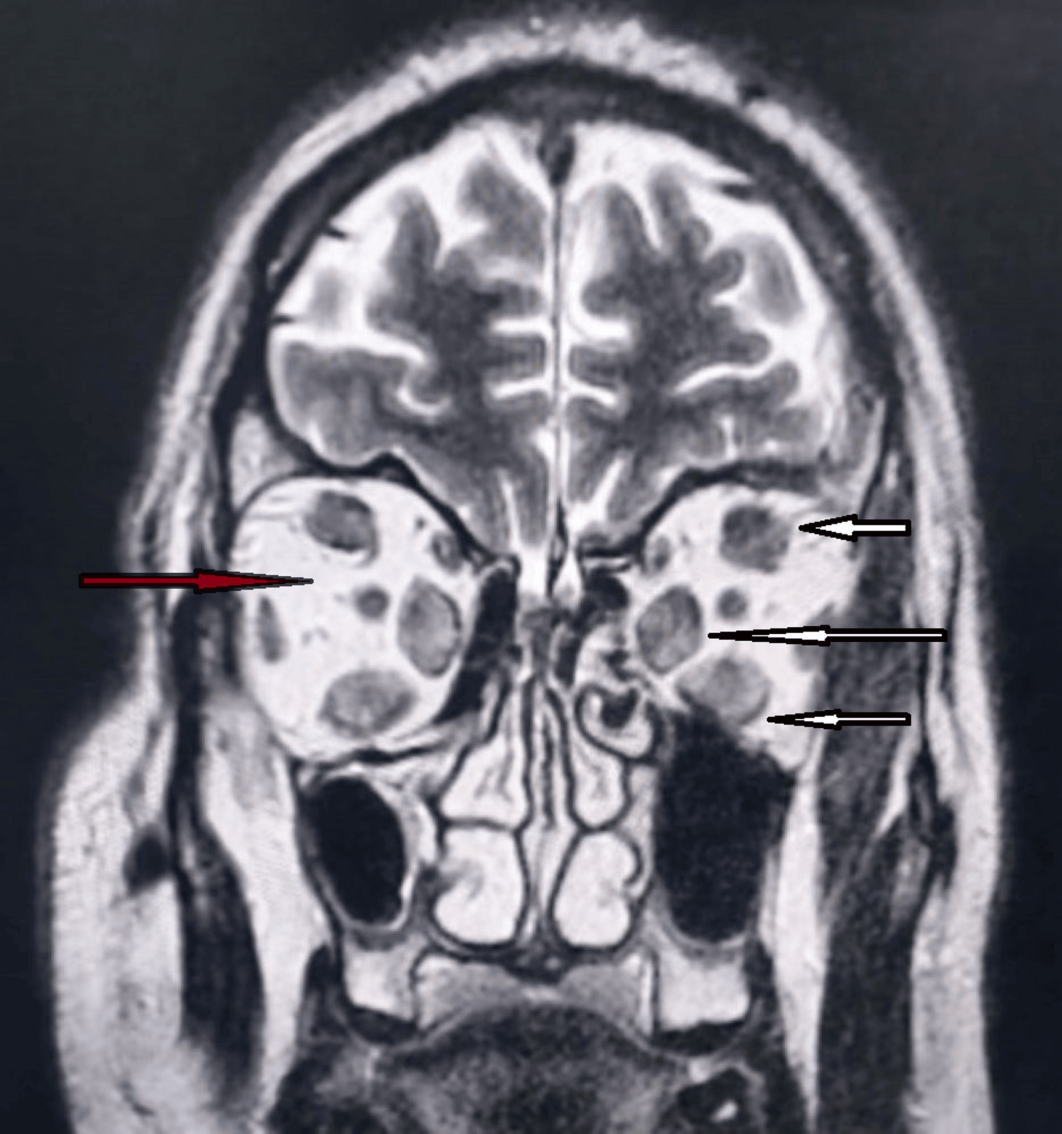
Orbital MRI coronal view showed an enlargement of the internal, inferior, and superior extraocular muscles in both eyes (white arrows) and orbital fat hypertrophy (brown arrow) Image provided by the ENT, head and neck surgery department of Hassan II University Hospital of Fez, Morocco, taken during consultation
